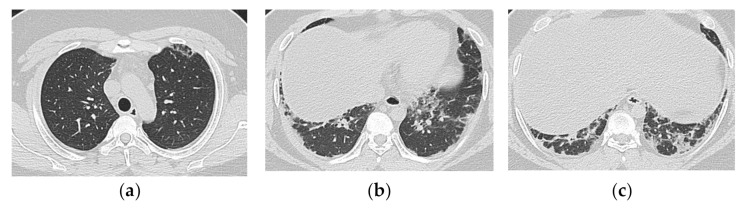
A 19-year-old man with interstitial lung disease associated with dermatomyositis (anti-MDA5 Ab positive). On HRCT (a–c), bilateral subpleural patchy consolidation are seen with basal predominance. Peribronchovascular component is not predominant. The onset was subacute, but the clinical course was stable.
